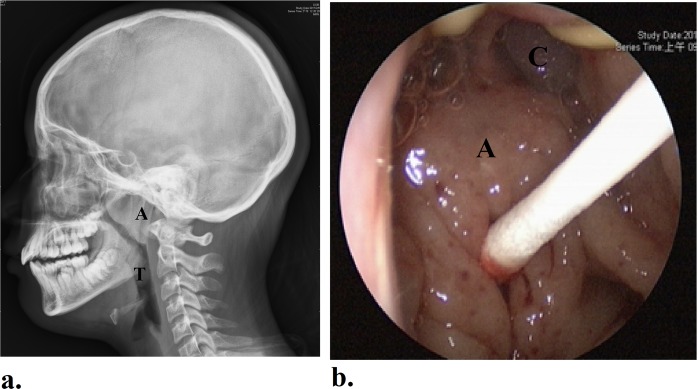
The size of nasopharyngeal adenoids was determined by skull lateral-view radiography (a). Nasopharyngeal colonization was determined by swabs for bacterial culture and pneumococcal PCR before removal of adenoid tissue during transoral endoscopic surgery. (b) A: adenoid; T: palatine tonsil; C: posterior nasal choanae.
